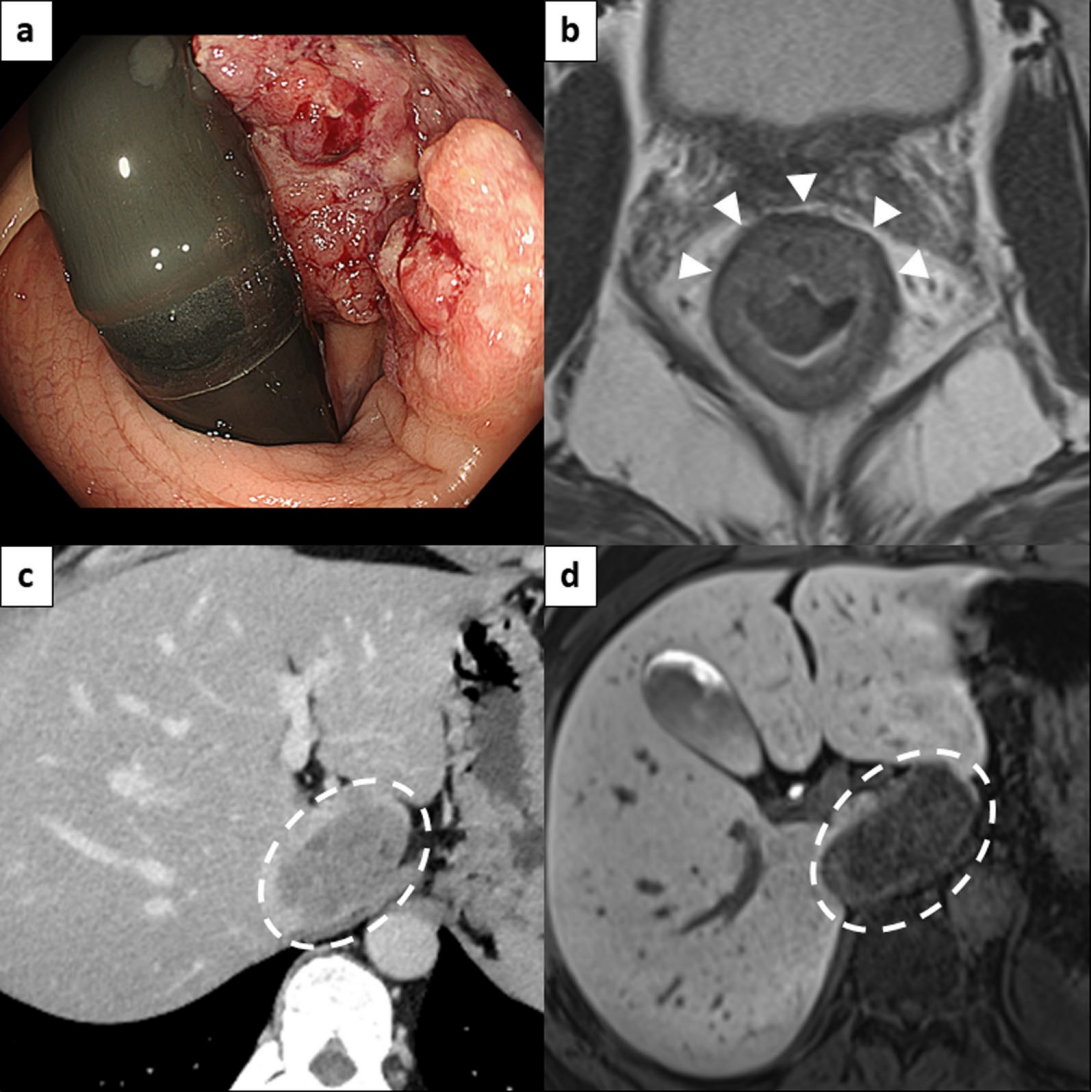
Imaging findings of primary and metastatic lesions before ICI therapy. a Inverted view of the endoscopic image at the rectum, showing a slightly elevated tumor located in the lower rectum. b Axial MRI sections of the rectal tumor, with arrowheads indicating a T2, EMVI-, CRM- rectal tumor. c CECT and d EOB-MRI of the liver, where the dashed line marks a 50 mm-sized solitary liver metastasis in the caudate
